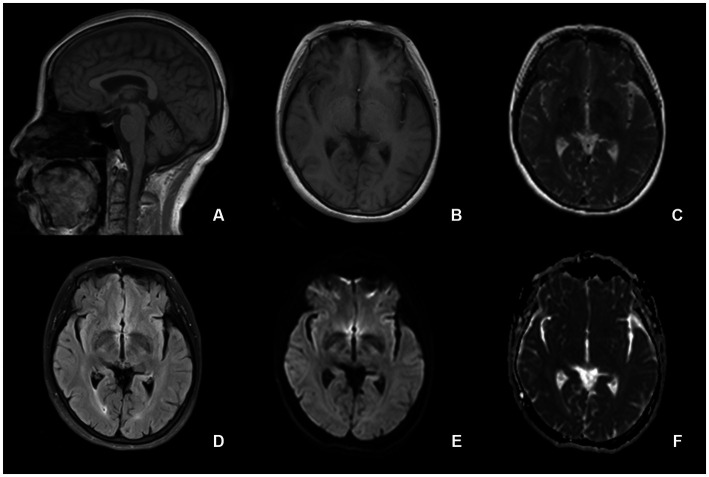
Brain magetic resonance imaging suggests normal structures. There are no midline structural abnormalities associated with circadian rhythms. (A) sagittal T1-weighted image, (B) axial T1-weighted image, (C) T2-weighted image, (D) fluid-attenuated inversion recovery, (E) diffusion-weighted imaging, and (F) apparent diffusion coefficient.
